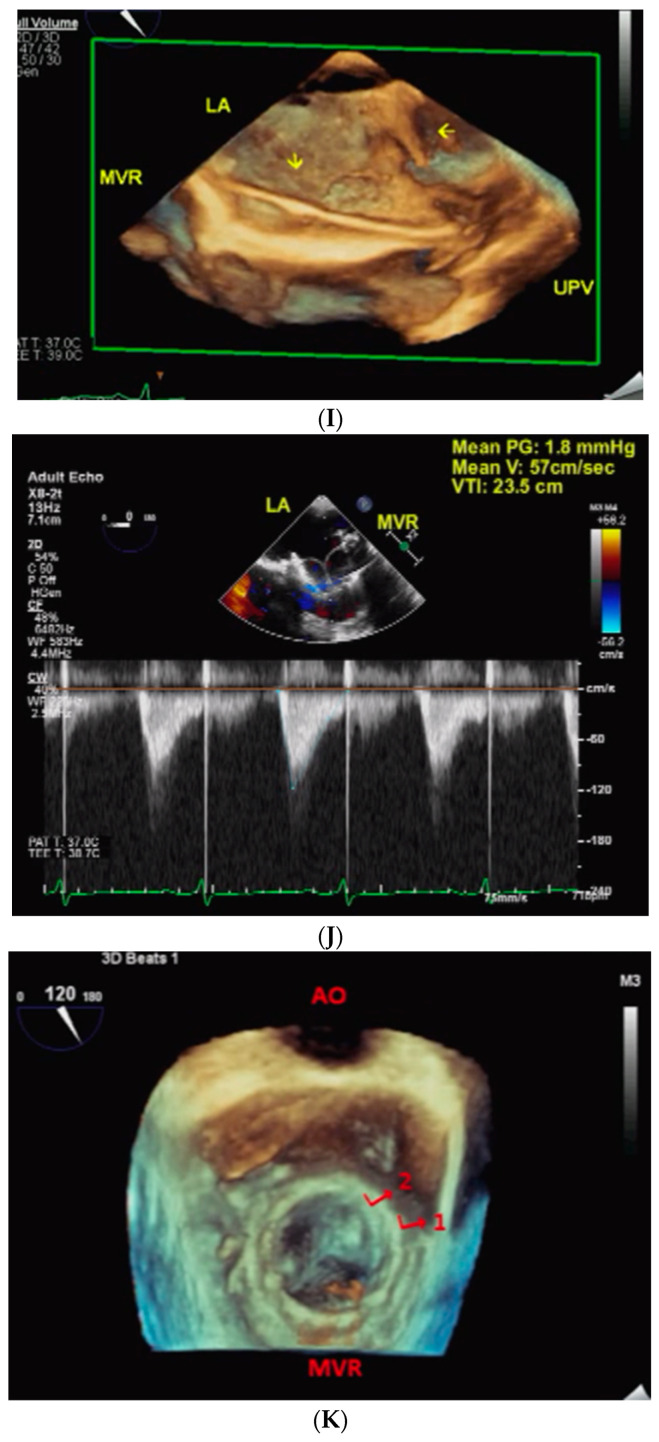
(A–K), Video S1. 78-year-old female with severe bioprosthetic mitral valve stenosis (Case 10). Pre-procedure. Two-dimensional transthoracic echocardiography with color Doppler guided continuous wave Doppler in (A) shows a mean pressure gradient (PG) of 14 mmHg across mitral valve replacement (MVR) consistent with severe stenosis. Two-dimensional transesophageal echocardiography (B,C) using the same technique as in (A) shows a mean pressure gradient of 13.1 mmHg across MVR also consistent with severe stenosis in (B). Turbulent flow across MVR as well as a thrombus (TH) in the left atrial appendage (LAA) and adjacent left atrium (LA) body are demonstrated in (C). Live/real-time three-dimensional transesophageal echocardiography (D–G, Video S1). The arrow in (D) points to the MVR orifice which measures 0.72 cm^2^ in multiplanar reconstruction (MPR) mode consistent with severe stenosis. Viewing the LA body en face by cropping the full volume dataset from below shows the TH (arrows) extending to near the MVR in (E). Video S1 shows both fixed (left arrow) and mobile (right arrow) components of the TH. The right upper panel in F shows the TH involving LAA almost completely and extending to the adjacent LA body (arrow). The arrow in the right lower panel shows the TH adjacent to the aorta (AO). The arrow in the left lower panel shows the TH adjacent to PA. The combined volume of the TH in LAA and adjoining LA body measured 19.4 mL as shown in (G). During Procedure. Two-dimensional transesophageal echocardiography (H). The lower arrow in H shows the catheter deployment device passing through MVR and lodged in the left upper pulmonary vein (UPV). The upper arrow points to the TH which was bypassed during the procedure. Live/real-time three-dimensional transesophageal echocardiography (I). The vertical arrow in (I) points to the catheter deployment device bypassing and avoiding the TH (horizontal arrow). Post-procedure. Two-dimensional transesophageal echocardiography (J). Color Doppler directed continuous wave Doppler examination in 4 chamber view in J shows a marked decrease in the mean pressure gradient across the new MVR to 1.8 mmHg indicative of relief of stenosis. Live/real-time three-dimensional transesophageal echocardiography (K). In (K), #1 and #2 represent the old and new prosthetic valve rings, respectively, in full volume short-axis view. LV = left ventricle; PA = pulmonary artery; RA = right atrium; RV = right ventricle; RVO = right ventricular outflow. PV = pulmonary valve.
